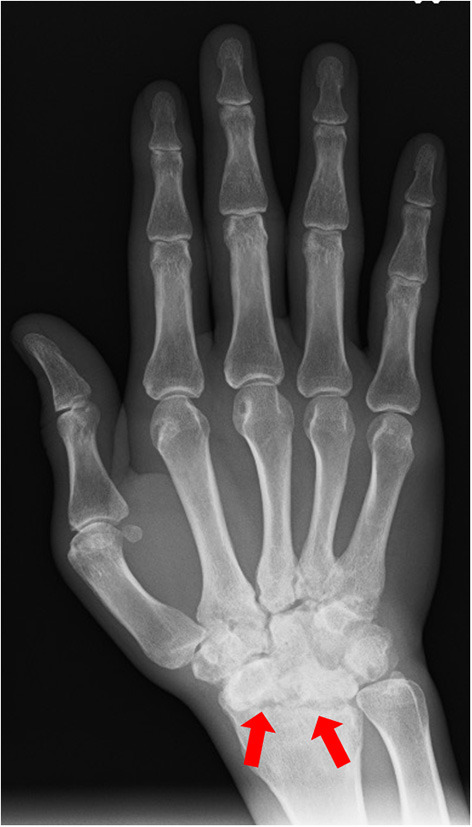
Radiography showing bone erosions of the wrist joint (red arrow) (image of right hand).
